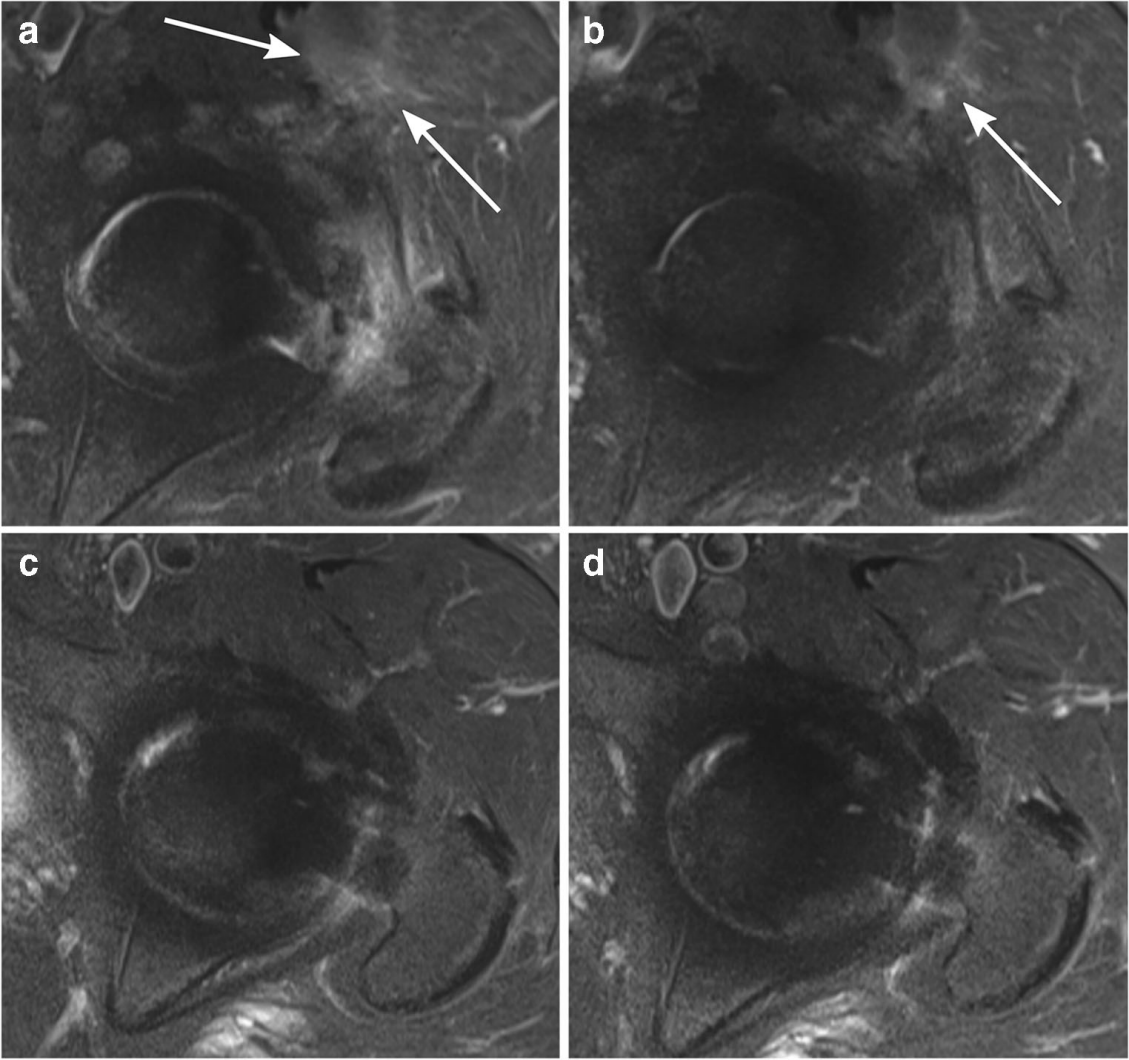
Periarticular soft tissue edema on axial STIR MRI. A 70-year-old asymptomatic woman with left-sided THA. Soft tissue edema (arrows) is present anteriorly along the surgical access route 3 months (a) and 6 months (b) after THA, which completely disappeared 12 months (c) and 24 months (d) after THA. *THA*, total hip arthroplasty
